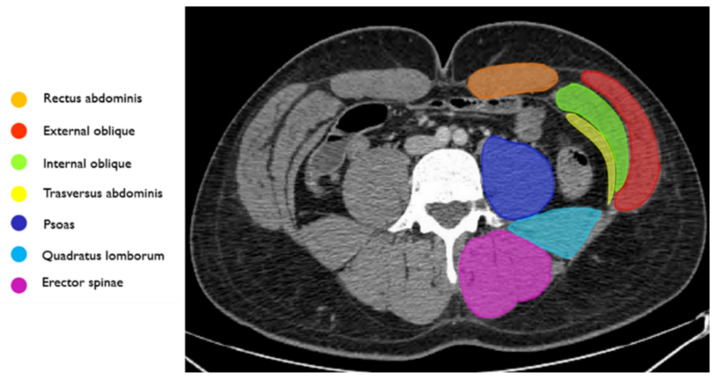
Annotated axial CT image of the abdominal wall musculature anatomy.
